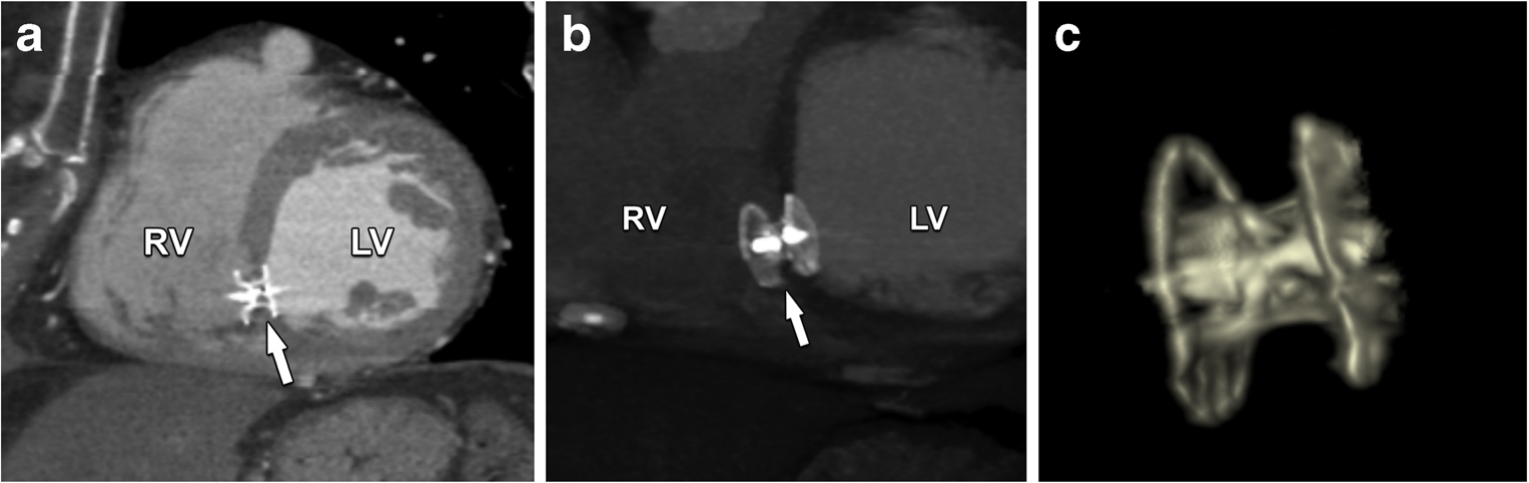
Two-chamber view of contrast-enhanced CT (a), maximum intensity projection (MIP) (b) and volume-rendered (c) images of a VSD closure device. *RV* right ventricle, *LV* left ventricle
